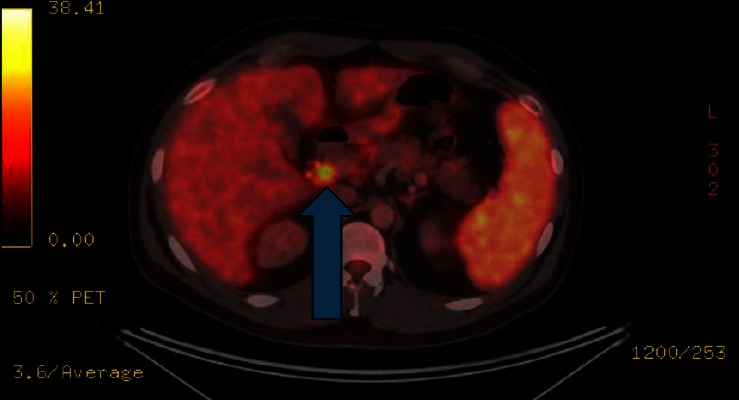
PET scan showing infradiaphragmatic lymphadenopathies and retroperitoneal intercavoaortic lymphadenopathies, measuring 12 × 10 mm, maximum SUV of 9.8.
